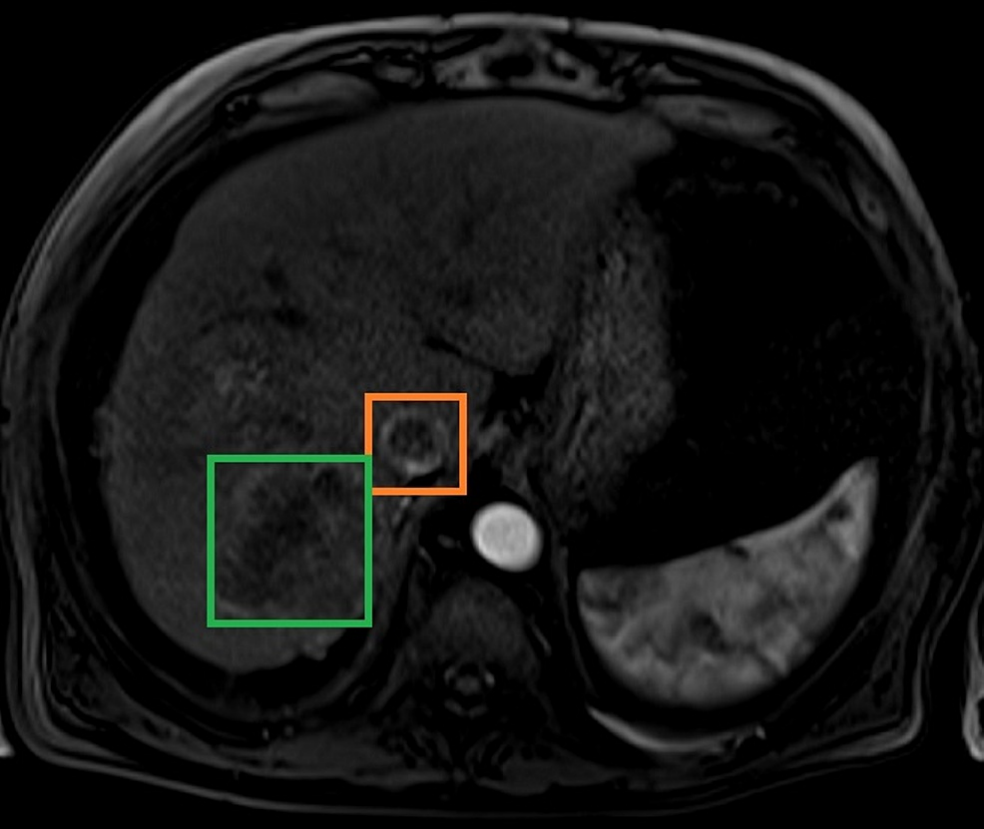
Abdominal MRI (T2) showing inferior vena cava with thrombus and hepatic tumor. Orange box: Inferior vena cava with thrombus; Green box: Hepatic tumor.
